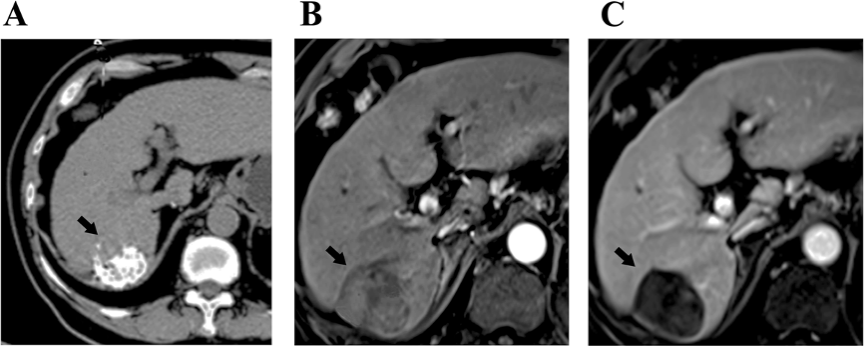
Radiofrequency ablation after transarterial chemoembolization was performed on the same patient with 6-cm HCC after initial TACE treatment. (A) CT scan after TACE treatment shows lipiodol uptake in the central aspect of the lesion. (B) Contrast-enhanced MRI scan at 4 weeks after RFA shows complete tumor necrosis without arterial enhancement within the lesion. (C) Contrast-enhanced MRI scan at 6 months after combination treatment shows no tumor recurrence in the liver.
